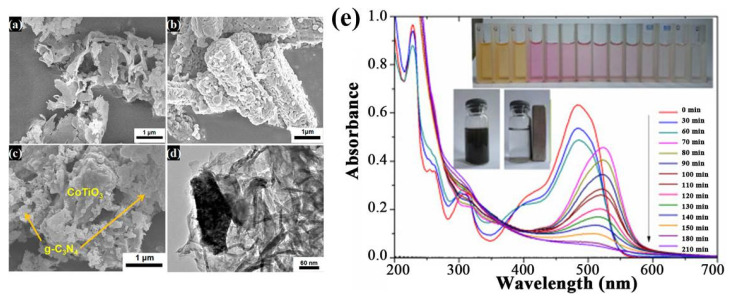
SEM images of (a) g-C_3_N_4_, (b) CoTiO_3,_ (c) CT-U and (d) 0.15% CT-U. Used with permission from [149]. Copyright 2016 American Chemical Society; (e) the photodegradation of Orange II by CuFe_2_O_4_@C_3_N_4_/H_2_O_2_/Vis system (inset: the solution before and after magnetic separation using an external magnet). Used with permission from [150]. Copyright 2015 Elsevier.
